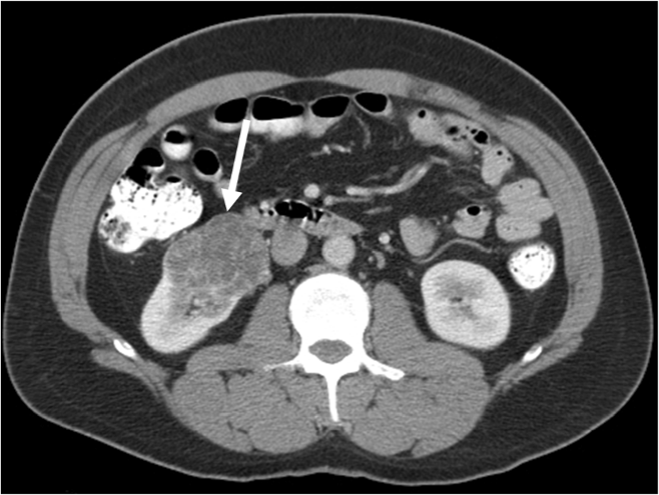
A 43-year-old male with Fuhrman grade 2 cystic clear cell renal cell carcinoma (ccRCC). Contrast-enhanced transverse CT image demonstrates an exophytic multilocular cystic mass in the inferior pole of the right kidney (arrow). Although multiple relatively thick enhancing septa are seen, no solid component is identified. This cystic ccRCC was categorized as Bosniak III.
